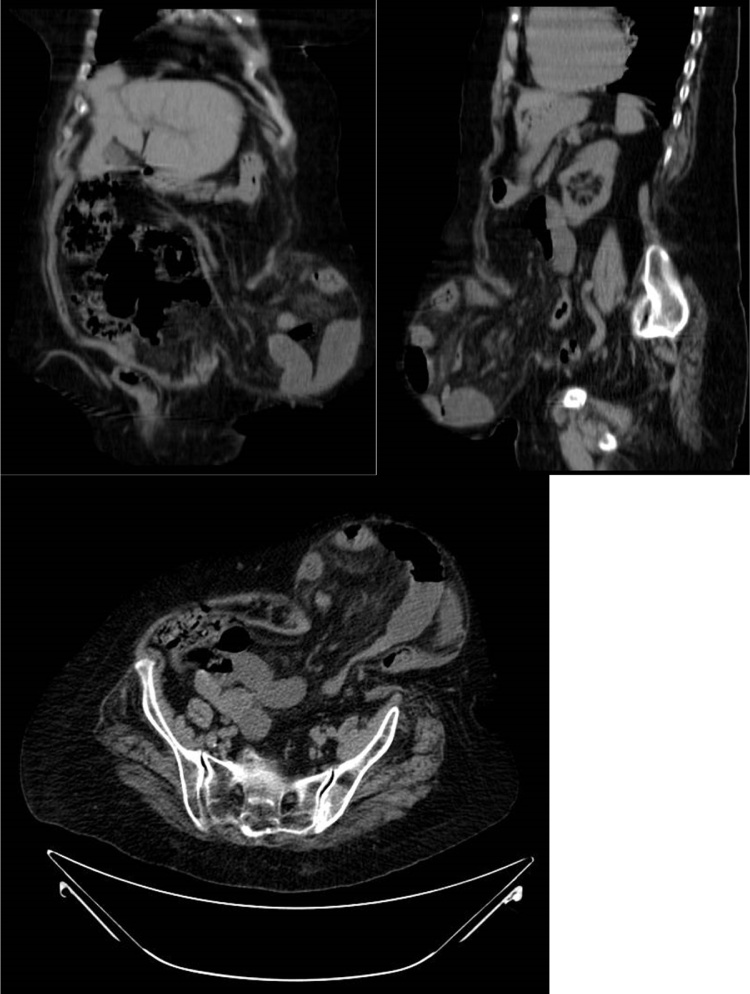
CT scan reveal several loops of small bowel introducing into a large defect (3 × 3 cm) on the left lateral abdominal wall, at the level of the iliac crest, and causing a SBO of the upper part.
